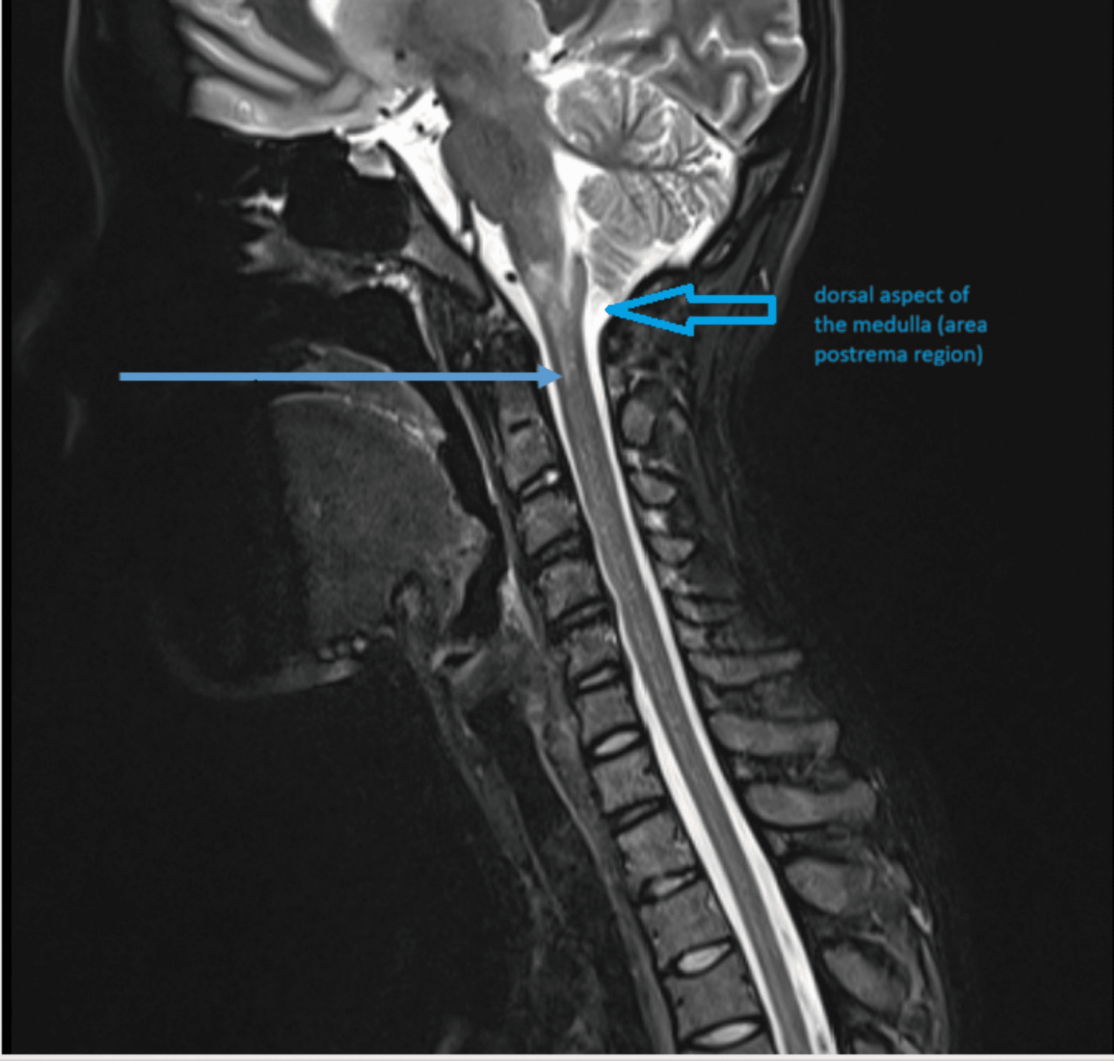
MRI spine: T2 hyperintense signal across the cervical medullary junction measuring nearly 2.7 cm in the craniocaudal extent centered along the floor of the fourth ventricle and in the central cord MRI: magnetic resonance imaging
